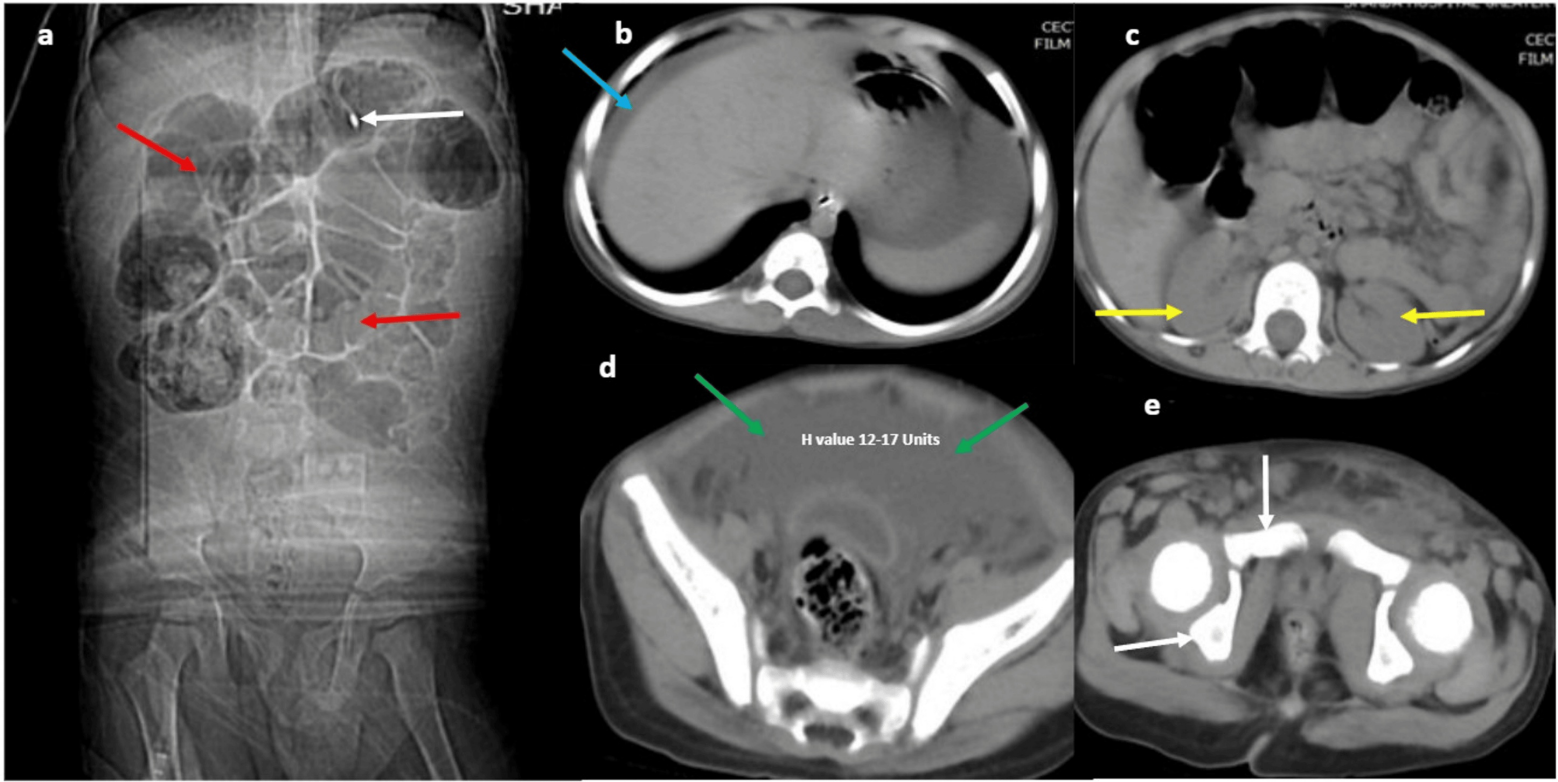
a-e: NCCT scan images of the abdomen and pelvis in a four-year-old male child with a very recent road traffic injury followed by abdominal pain, distension, anuria, and suspected urinary bladder injury. The scout view (a) shows the nasograstic tube in situ (white arrow), with diffusely dilated small and large bowel loops (red arrows in a). The upper abdomen (b) shows free peritoneal fluid around the hepatic capsule (blue arrow in b). Both kidneys and perirenal tissues appear normal (yellow arrows in c). Extensive free fluid, with attenuation of 12-17 HU, is seen in the pelvis (green arrows in d). The pubic bones and iliac bones (white arrows in e) appear normal. NCCT: non-contrast computed tomography; HU: Hounsfield Unit
